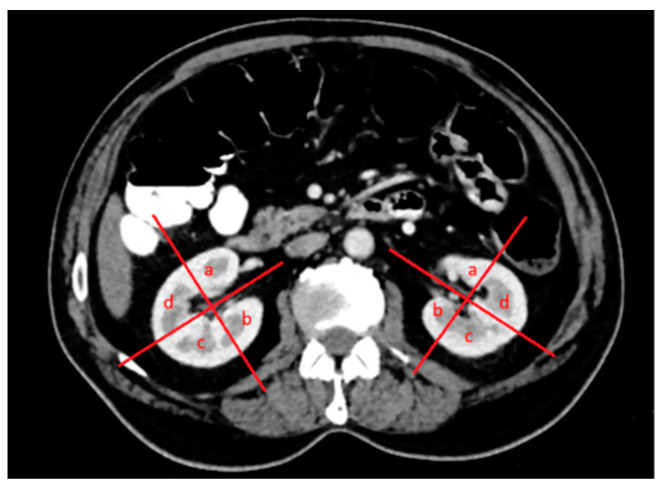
Both kidneys in the axial venous-phase computed tomography (CT) image were divided into four quadrants in the upper, middle, and lower poles, with a line drawn parallel to the vascular structures originating from the hilus-collecting system and a second line perpendicular to it: (a) anteromedial, (b) posteromedial, (c) posterolateral, and (d) anterolateral.
